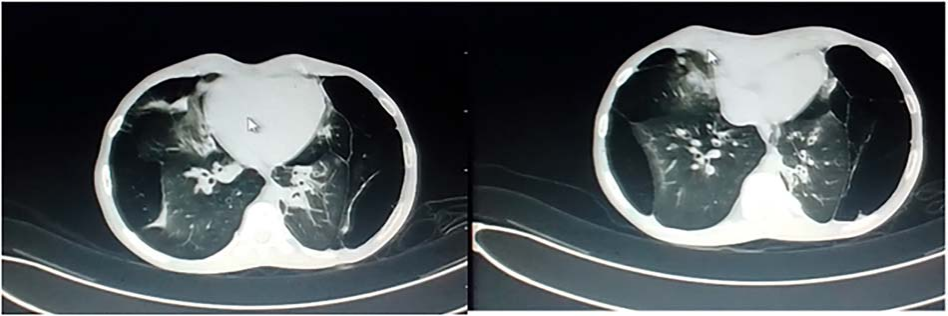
Axial view of CT scan of the chest showed multiple cystic lesions of varying sizes, with thin walls are seen in both lungs.
